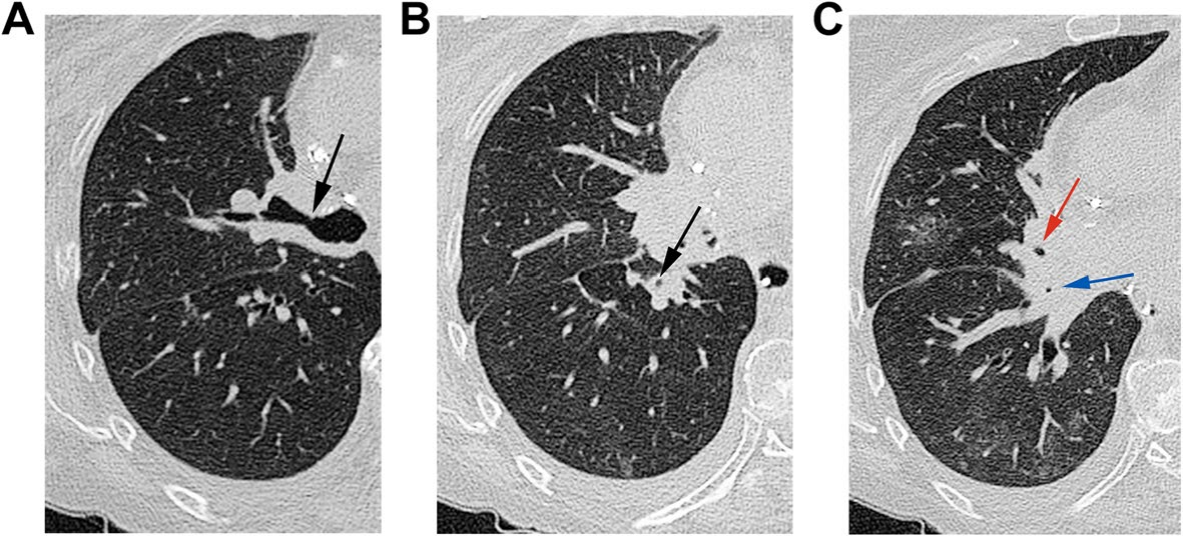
Multiple non-anastomotic bronchial stenoses in a 61-year-old female who underwent bilateral lung transplants for alpha-1 antitrypsin deficiency. A Axial CT image (lung window) shows narrowing of the proximal right upper lobe bronchus (arrow). B Axial CT image (lung window) shows narrowing of the bronchus intermedius (arrow). C Axial CT image (lung window) shows narrowing of the right middle (red arrow) and lower lobe (blue arrow) bronchi. Stents were subsequently placed in the right lobar bronchi, as well as the left mainstem and lobar bronchi (stenoses in these not shown)
